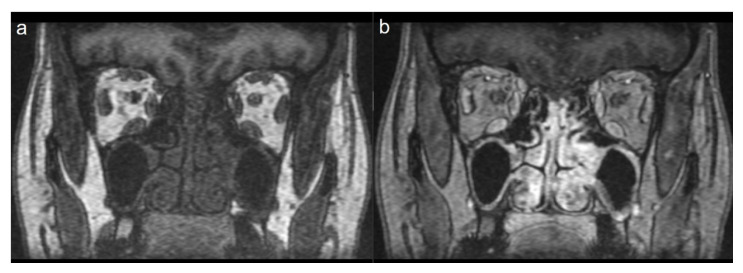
Coronal pre- (a) and postcontrast (b) T1-weighted image showing thickening and enhancement in the bilateral olfactory cleft and mucosa.
